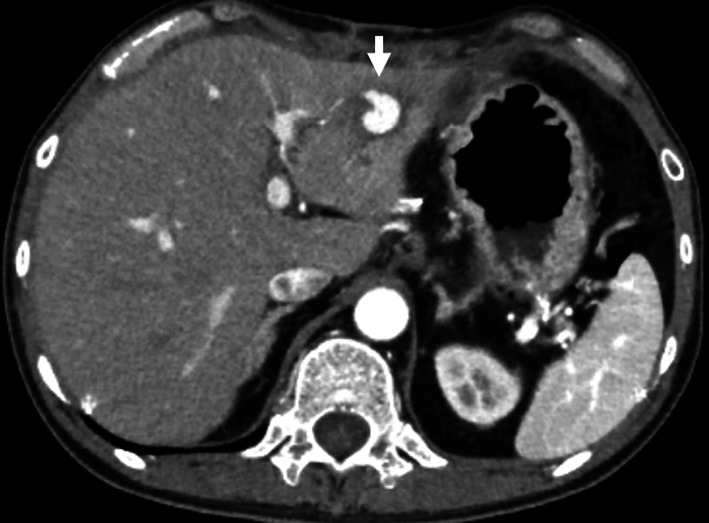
Computed tomography on postoperative day 25 revealed a hepatic pseudoaneurysm in the lateral segment of the liver. The white arrow indicates the hepatic pseudoaneurysm
